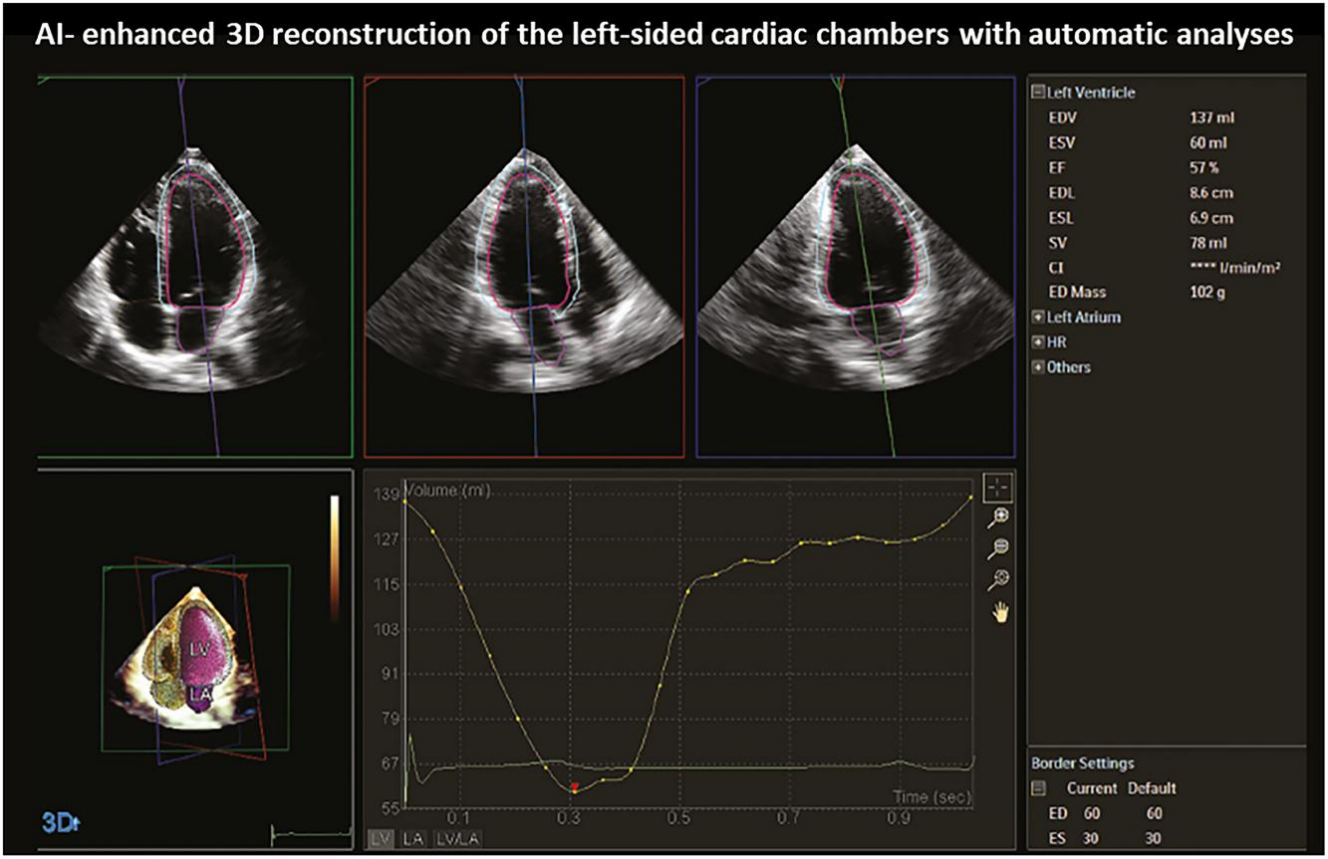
Example of AI-enhanced functional analysis with echocardiography. AI-enhanced automatic 3D reconstruction of the left side of the heart providing quantitative data, including end-diastolic volume, end-systolic volume, EF, and other measurements critical for assessing cardiac function.
